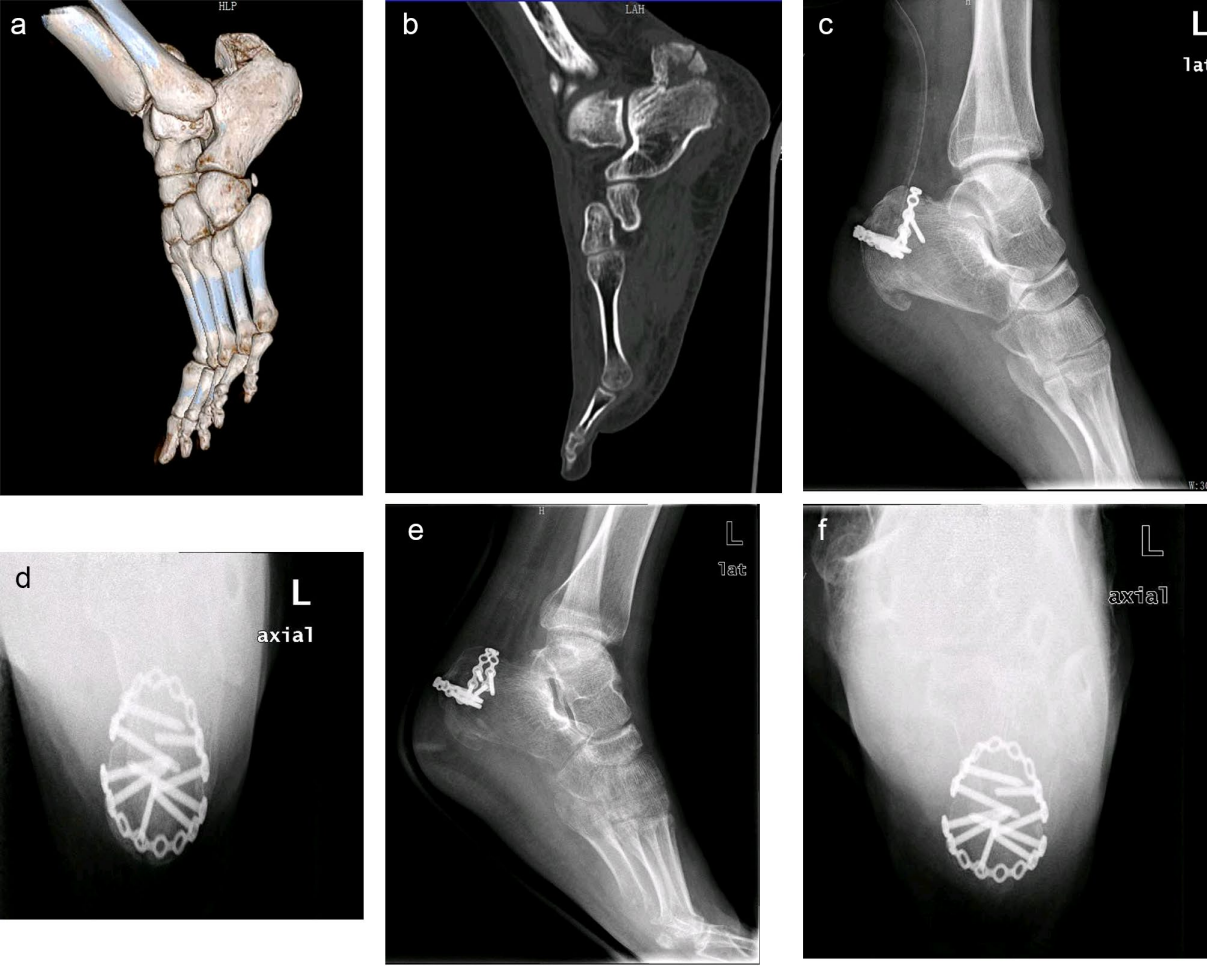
a.b: CT scan of the calcaneus plus three-dimensional reconstruction, the fracture of calcaneal tuberosity with obvious displacement. c.d: Lateral and axial films of calcaneus 3 days after operation. Two micro locking plates were used to fix the fracture block from the back of Achilles tendon insertion point and in front of Achilles tendon insertion point respectively. The reduction of fracture block was good and the internal fixation was firm. e.f: Lateral and axial films of calcaneus half a year after operation. The fracture healed well without complications
